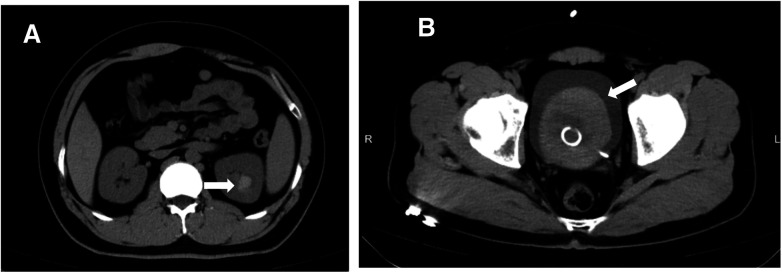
Postoperative CT showing blood clot formation. (A) Coronal non-contrast CT image revealing a hyperdense blood clot within the left upper pole calyceal diverticulum. (B) Coronal CT image showing a large intravesical blood clot occupying the bladder, with an indwelling ureteral stent visible *in situ*.
